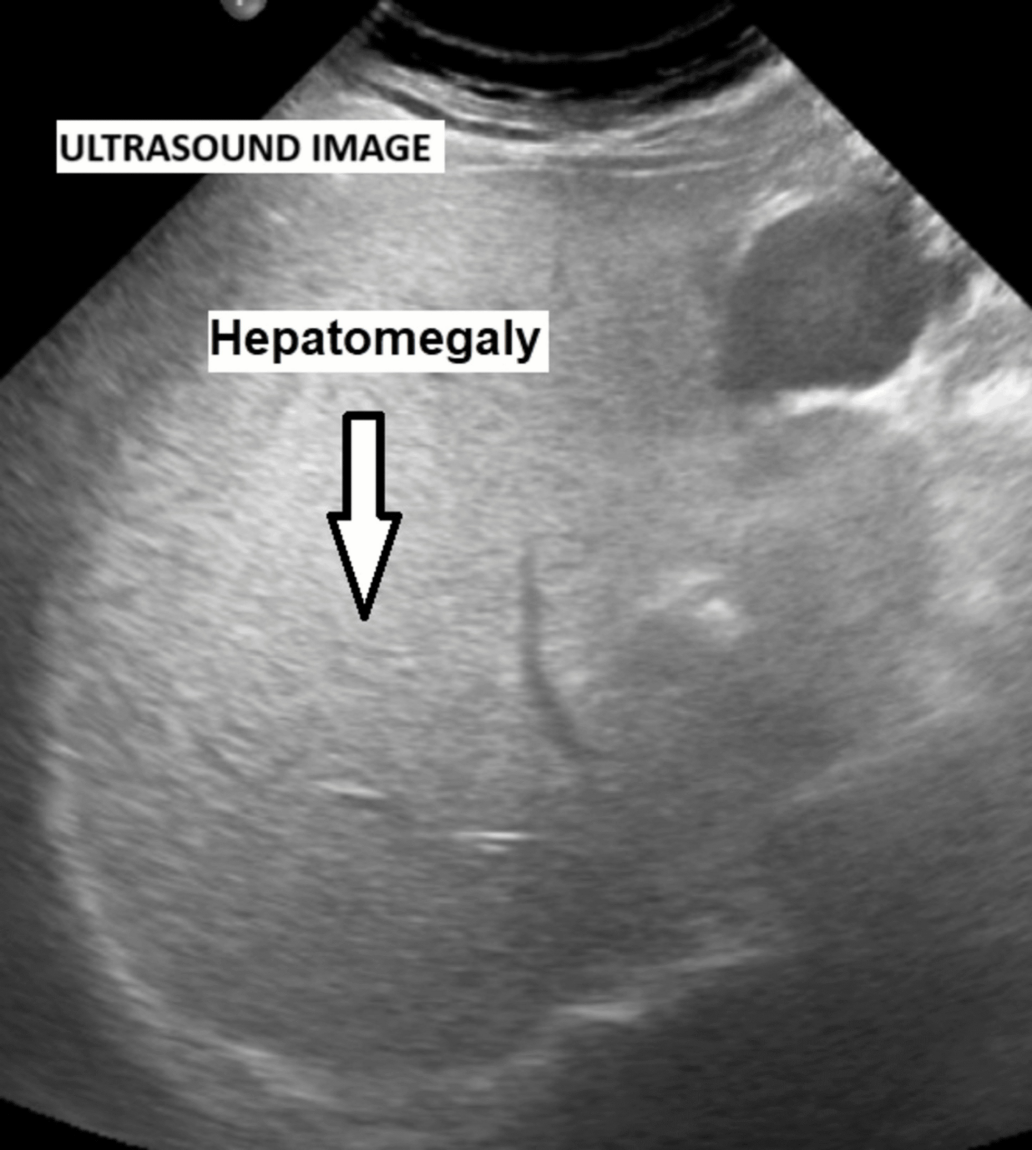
Abdominal ultrasound scan showing hepatomegaly
